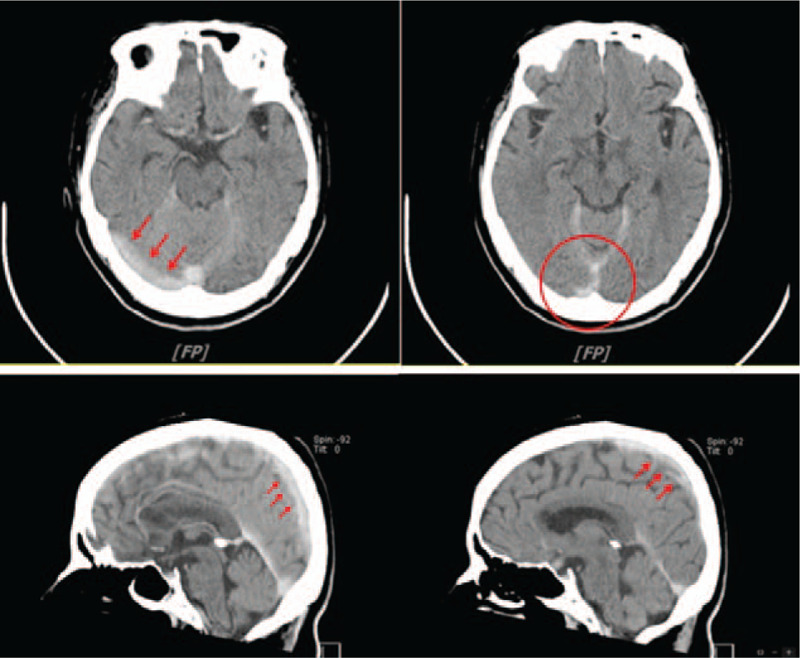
CT scans revealing CVT. CT = computed tomography, CVT = cerebral venous thrombosis.
